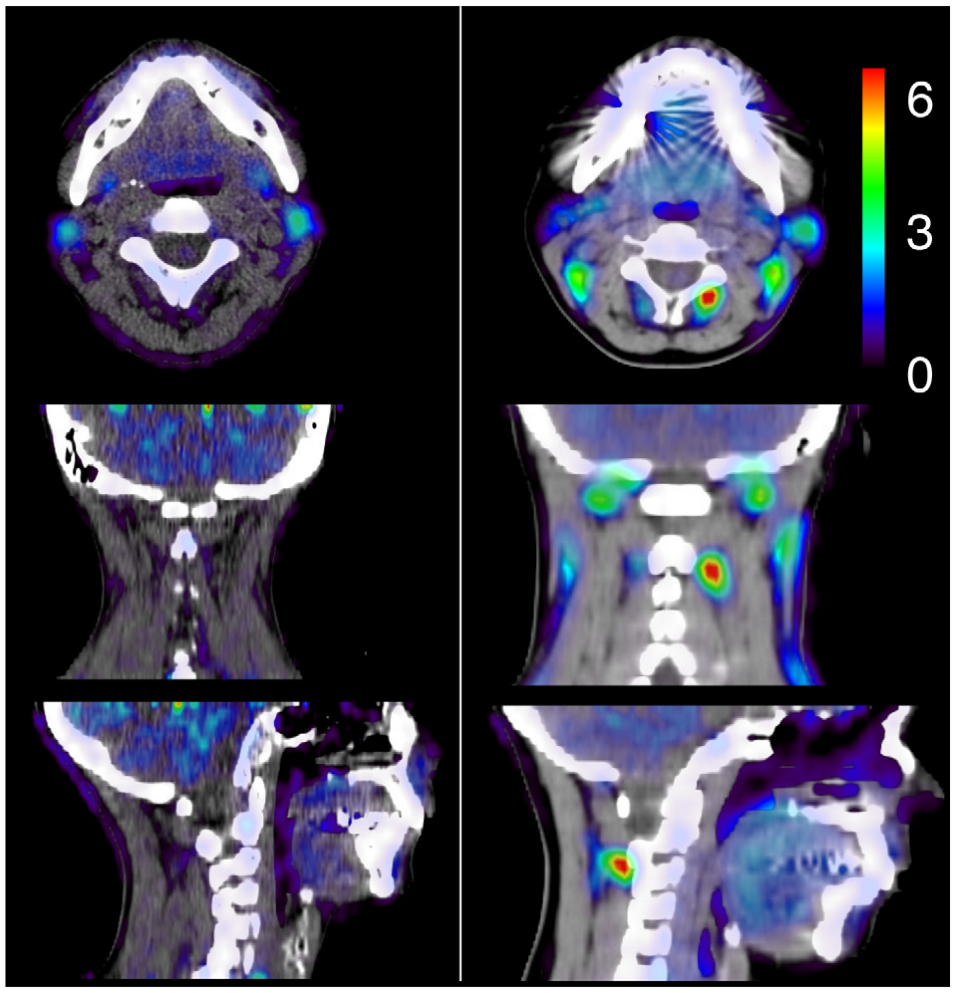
DDE uptake in a representative healthy control (left) and a whiplash patient (right). The patient displays high DDE uptake in the adipose tissue right of the spineous process of C2. PET images are overlaid on the subject's individual CT anatomy and tracer uptake is expressed as standardized uptake values.
